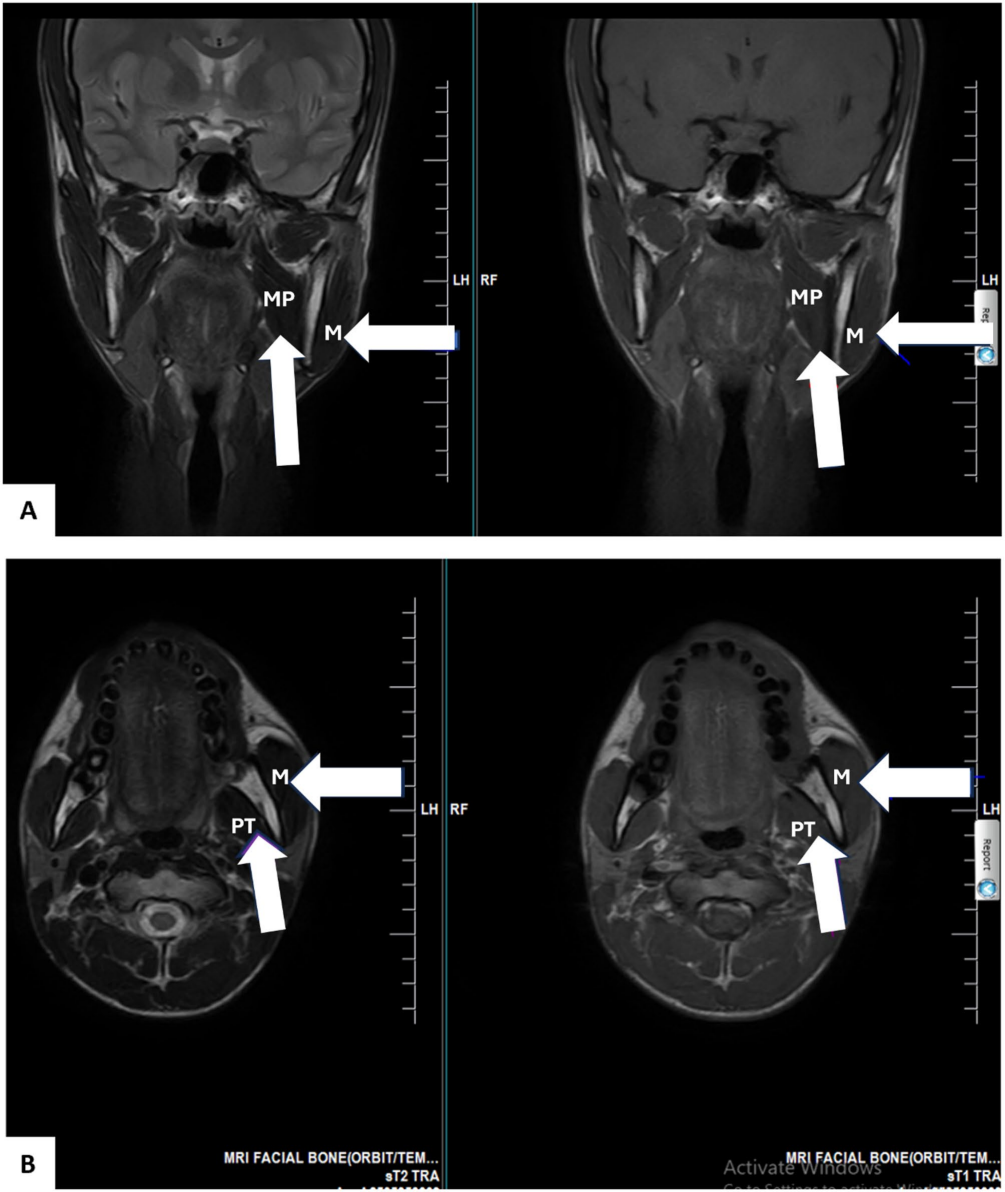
Muscular hypertrophy secondary to mandibular asymmetry. A Coronal T2-weighted (Left) and T1-weighted (Right) images showing significant hypertrophy of the left masseter muscle (white arrowhead, M = Masseter) and left medial pterygoid muscle (white arrow, MP = Medial Pterygoid). B Axial T2-weighted (Left) and T1-weighted (Right) images showing the increased bulk of the left masseter muscle (white arrowhead, M = Masseter) and left pterygoid muscles (white arrow, PT = Pterygoid) when compared to the right side
